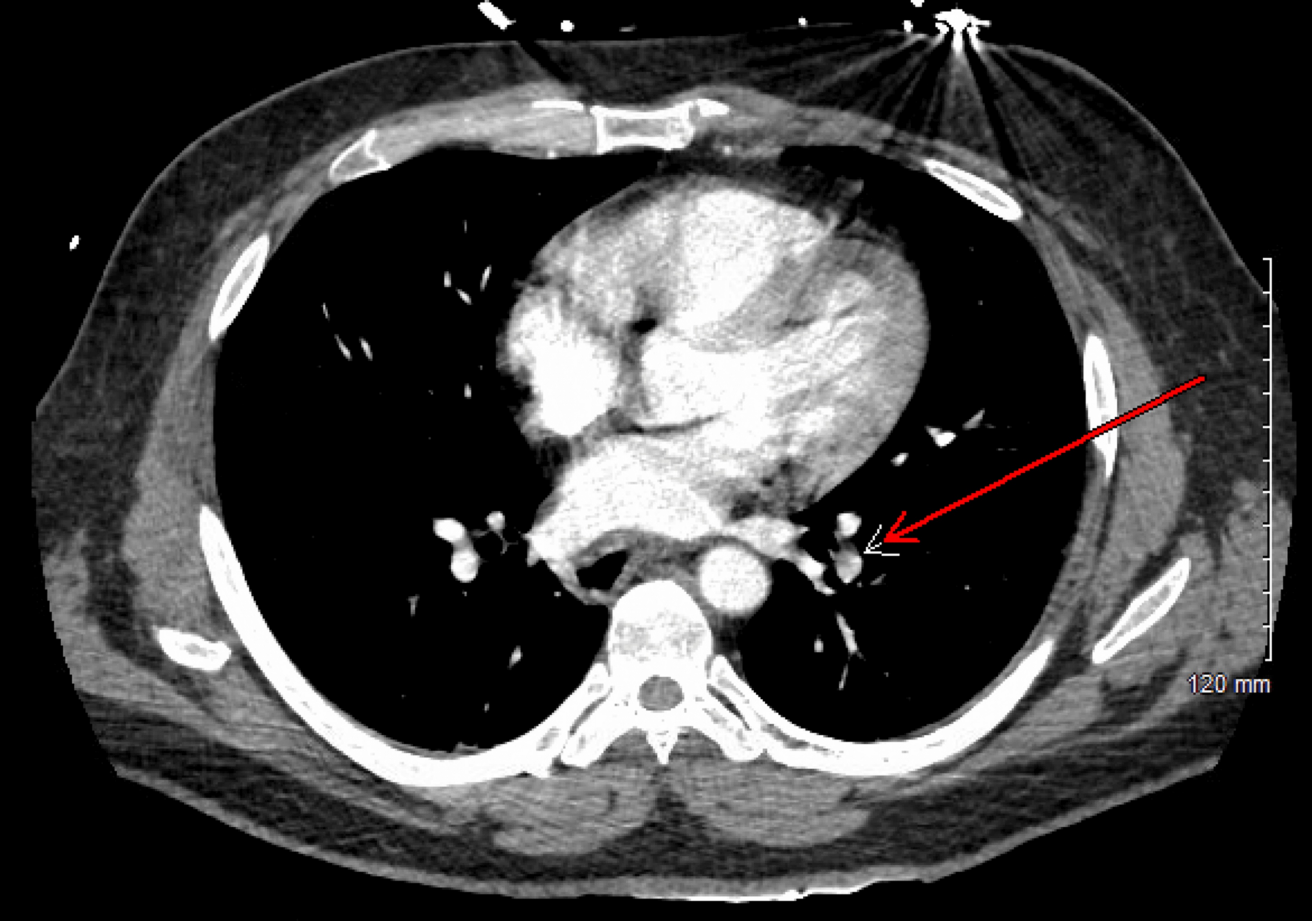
CTPA of the chest showing pulmonary embolism, pointed by a red arrow CTPA: CT pulmonary angiography
